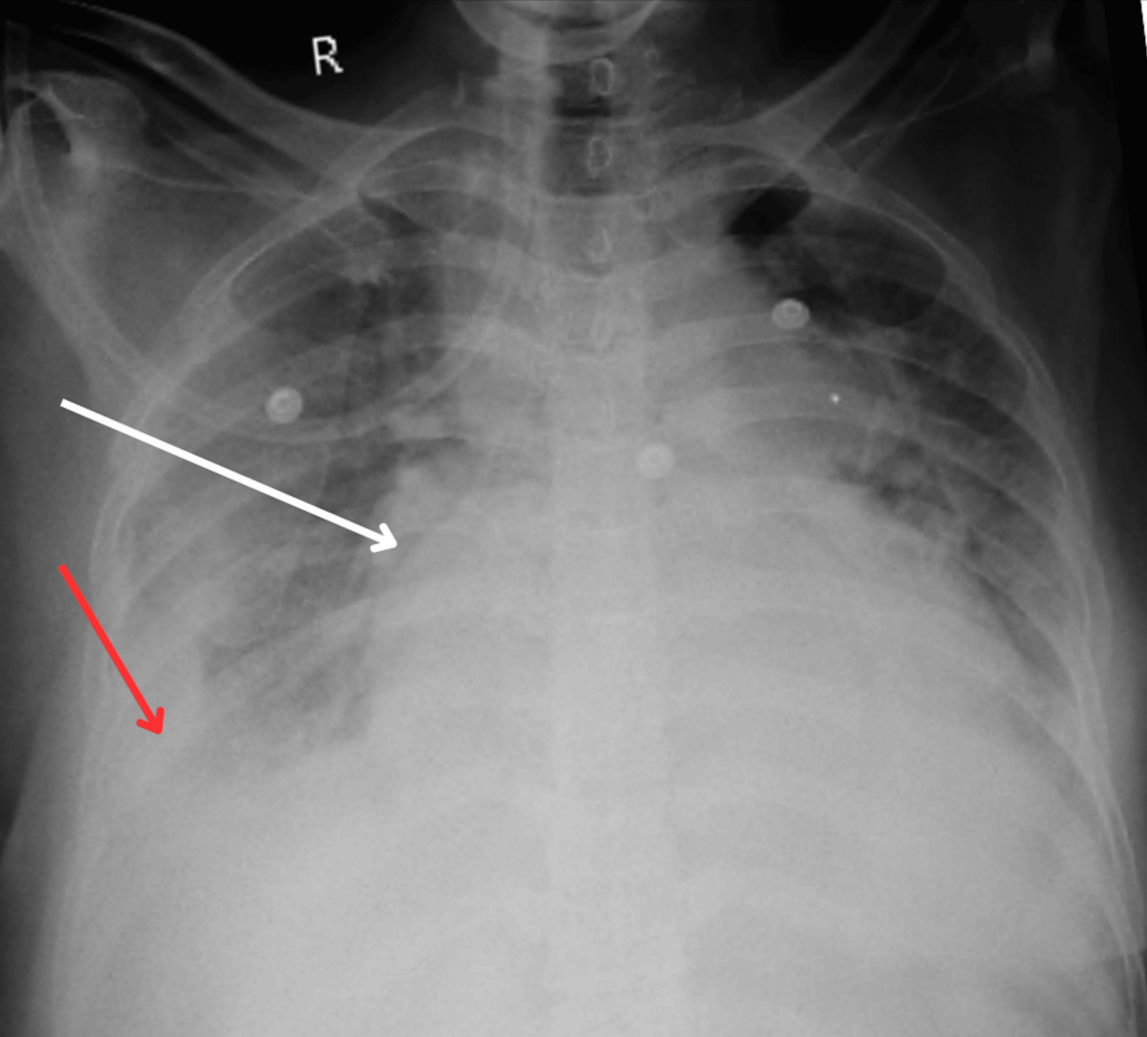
Chest X-ray showing pericardial effusion (white arrow) and pleural effusion (red arrow)
